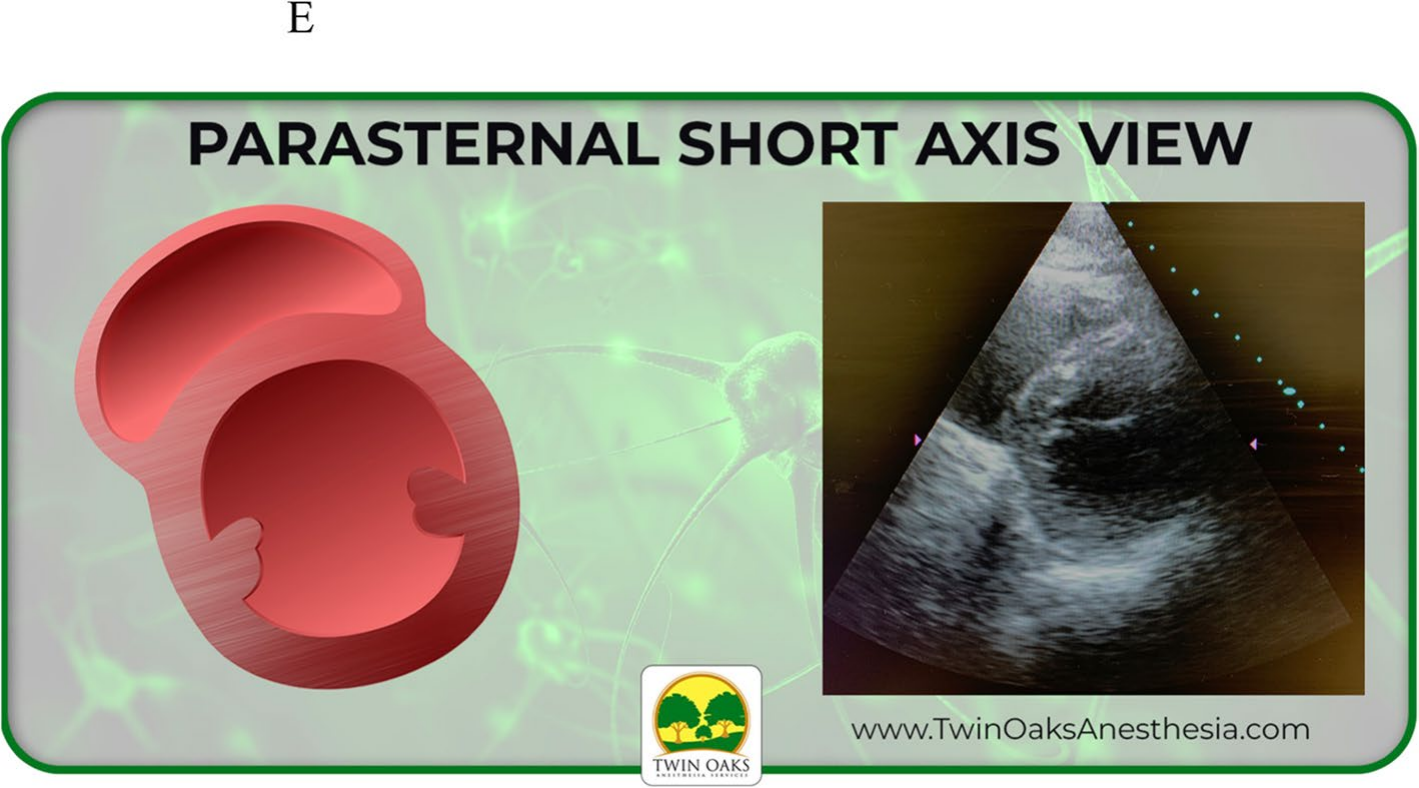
Study graphic of parasternal short axis graphic and corresponding TTE view
